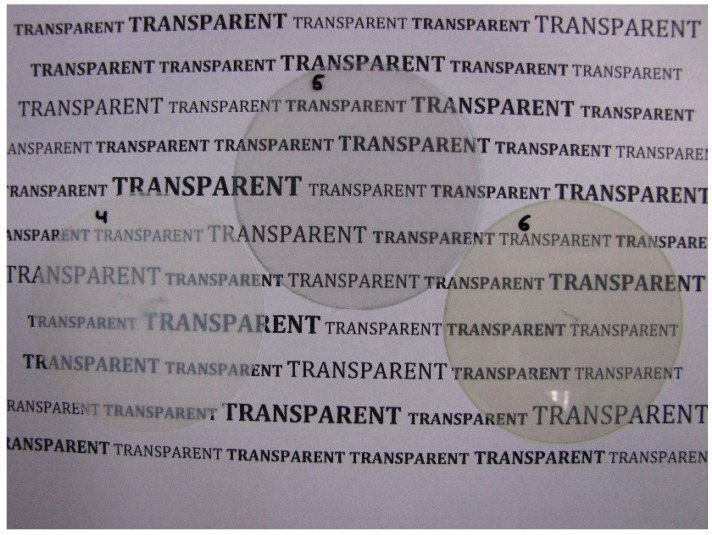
PET films spin-coated with three different TCO coatings. The left sample (4) is prepared with the AZO particles, the one in the middle (5) with ITO particles delivered as dispersion and the right one (6) with ITO powder dispersed by ultrasonic treatment.
